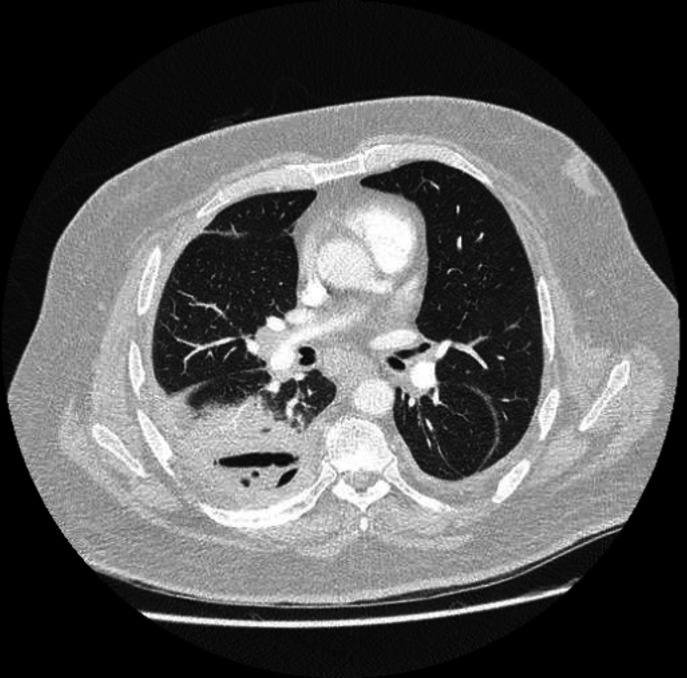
Axial CT image of the chest demonstrating right-sided lung abscess and empyema.
